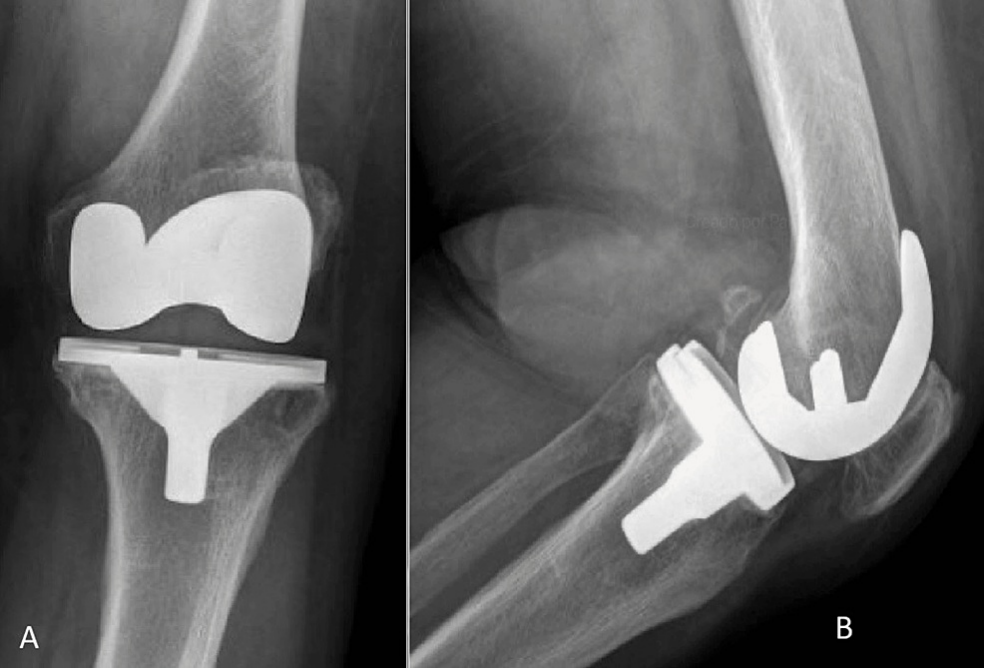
Anteroposterior (A) and lateral (B) radiographs of the primary total knee arthroplasty.
